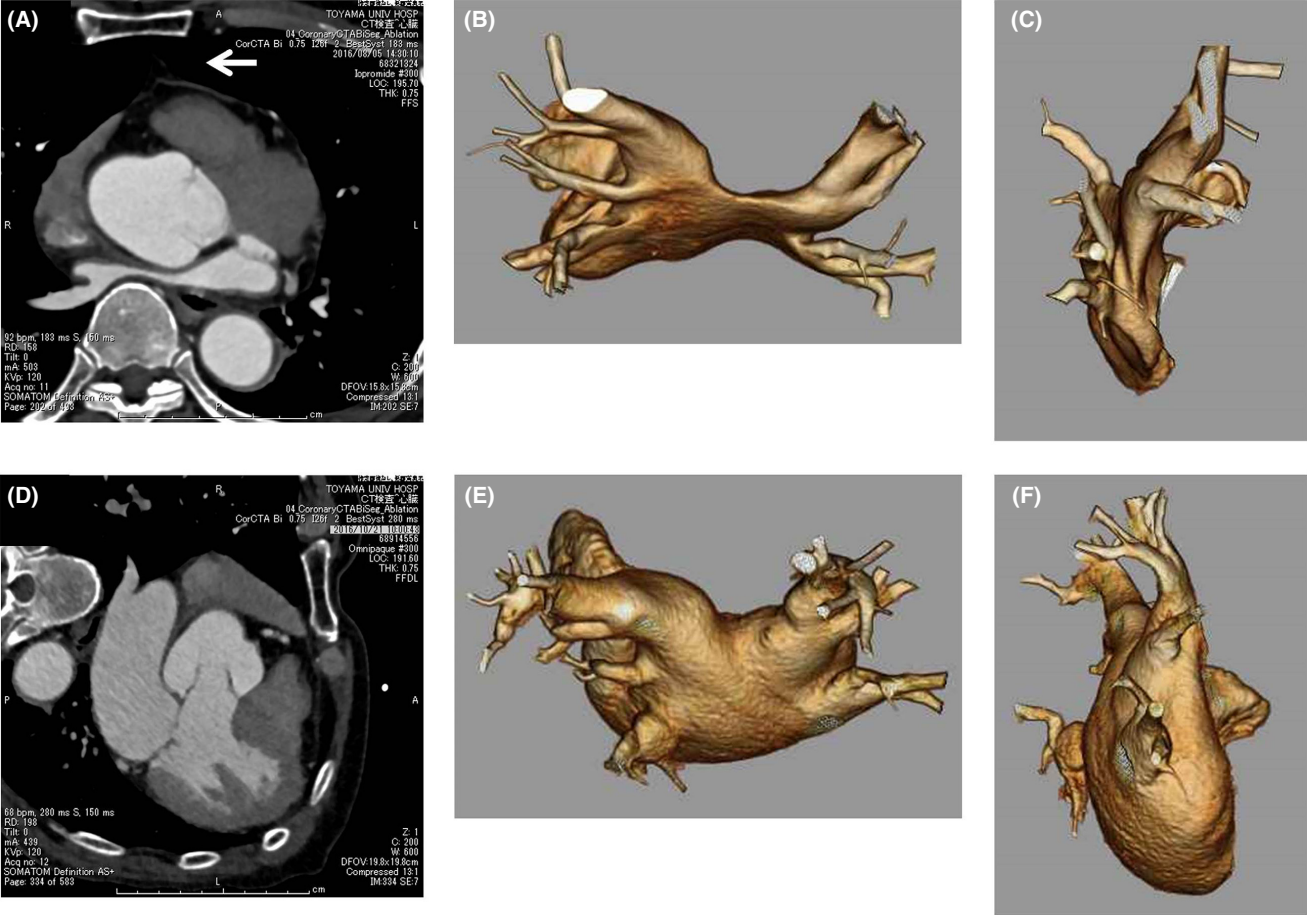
Enhanced chest computed tomography in supine position (A, B, C) and left lateral decubitus position (D, E, F). (A) There was a space in front of the heart (arrows) because of backward shift of the heart in supine position. Left atrium was compressed by mildly enlarged aortic root (maximum aortic root diameter was 41 mm) and the vertebra. Superior view (B) and right lateral view (C) of the left atrium showed extremely small left atrial dimension. (D) Compression of left atrium was relieved because of frontward shift of the heart in left lateral decubitus position. The space in front of the heart disappeared. Superior view (E) and right lateral view (F) of the left atrium showed normal left atrial dimension.
